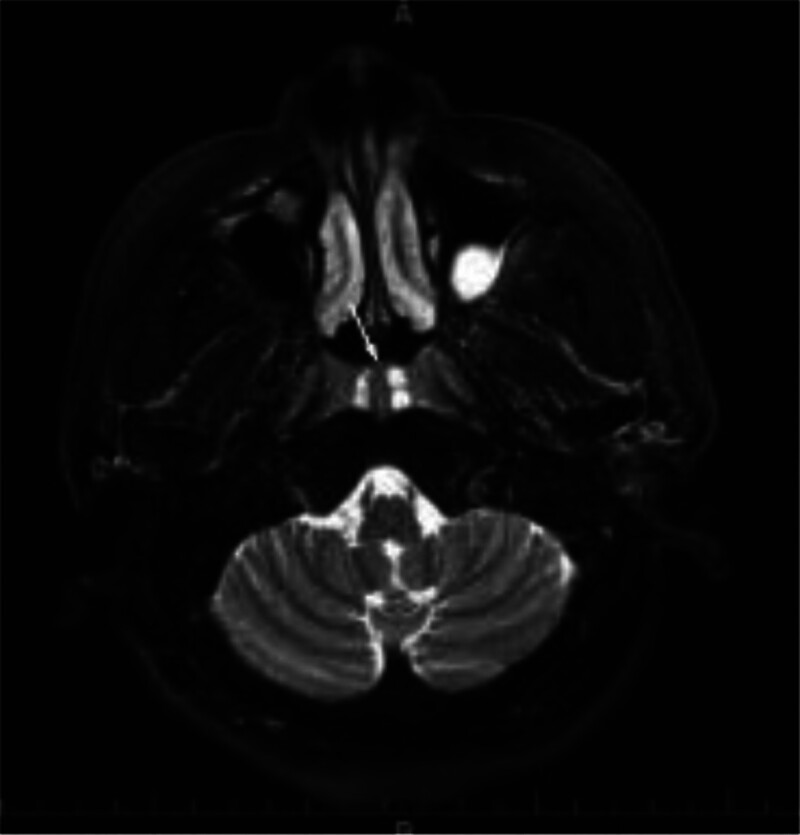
Nasopharyngeal MRI: white arrow indicate 3 nodular lesions with long T2 signal intensity. MRI = magnetic resonance imaging.
